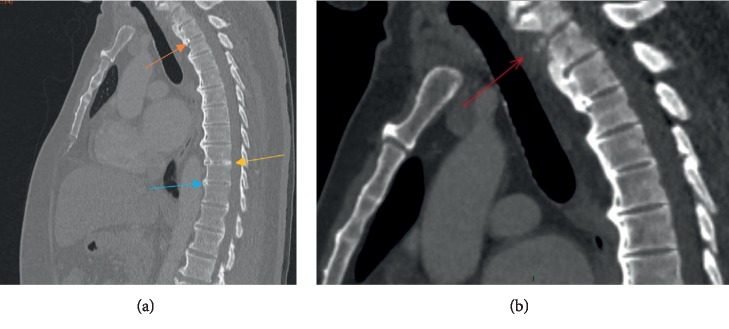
CT scan of the cervical and dorsal spine showing erosions on the anterior aspects of the lower vertebral endplate of C7 and the upper vertebral end-plate of T1 with a calcification located in between, at the anterior part of the C7-T1 intervertebral disc ((b) red arrow). (a) Calcifications were also present in the T2-T3 (orange arrow), T9-T10 (yellow arrow), and T10-T11 (blue arrow) intervertebral discs.
